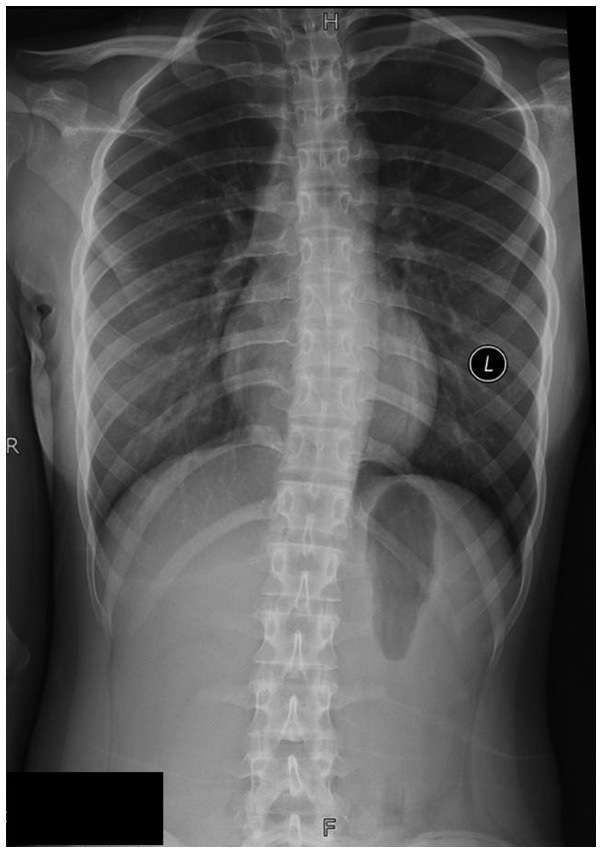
X ray revealing the presence of scoliosis in the spine plain film.
